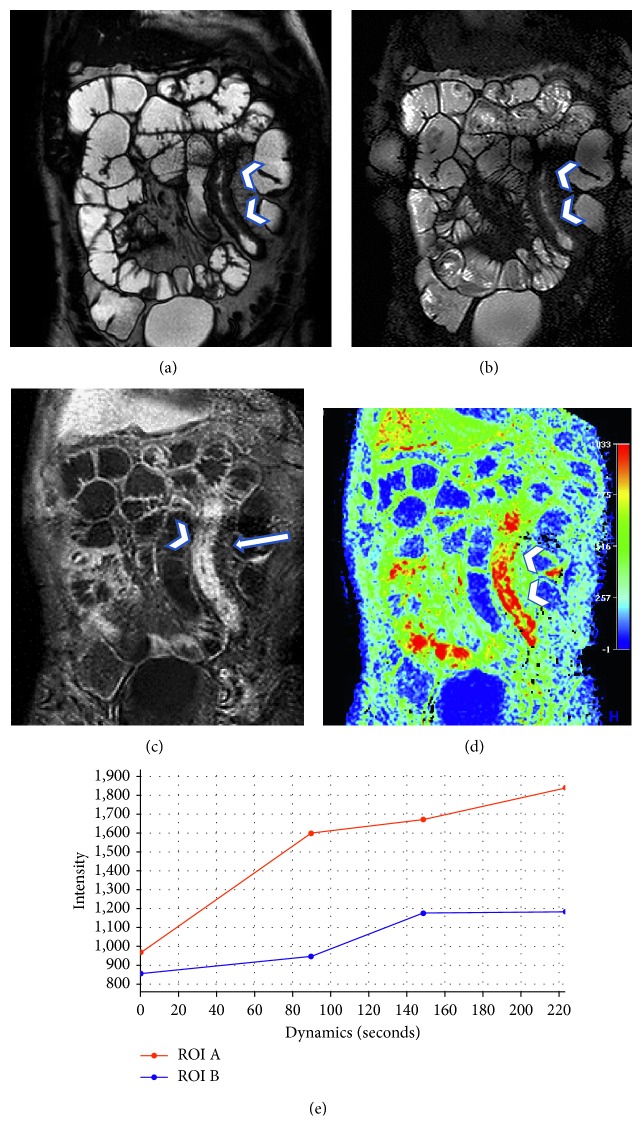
A case presenting with findings that do not allow classifying the disease as active or chronic; on the basis of the morphological sequences alone, there is an evident thickening of bowel ileal loop, not presenting hyperintensity in T2-weighted sequences (arrowhead in (a) and (b)), but with a slight vascular congestion (arrow in (c)) and a strong enhancement in dynamic study (arrowhead in (c)), that does present a very typical layered enhancement. The perfusion analysis demonstrates a time-intensity curves with an increasing trend of enhancement and higher perfusion values than those of the normal bowel, suggesting the presence of acute disease.
